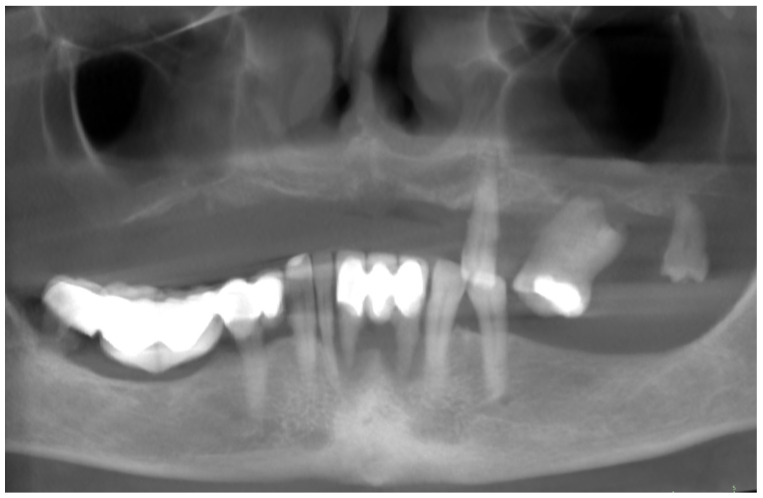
An orthopantomogram (OPG) view obtained using CBCT, showing the initial situation and signs of periodontitis (stage IV grade C). The residual dentition in the maxilla and advanced bone loss (almost 100% of the root’s length) around the incisors in the mandible are visible.
